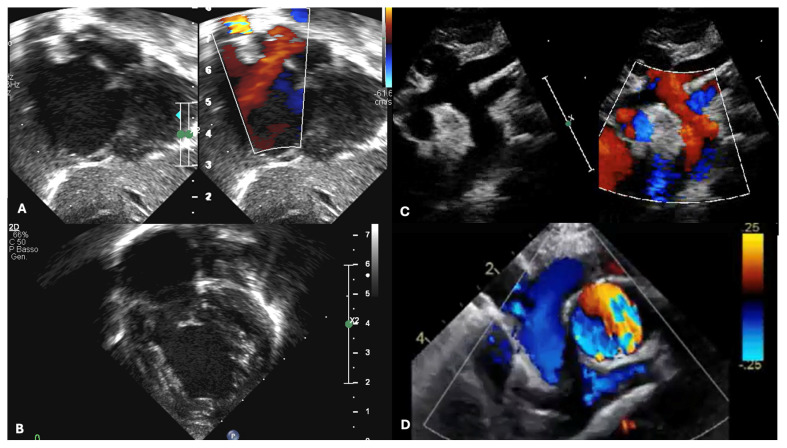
TTE evaluation focused on different cardiac structures that are subject to changes in the various phases of palliation. (A) Subcostal view demonstrating a PFO with a left-to-right shunt of a neonate with HLHS. (B) An apical 4-chamber view of a neonate with HLHS. (C) aortic arch in HLHS (D) Imaging of the superior cavo-pulmonary anastomosis from a suprasternal frontal view in a patient with Glenn: the SVC has been cut and connected to the right PA.
